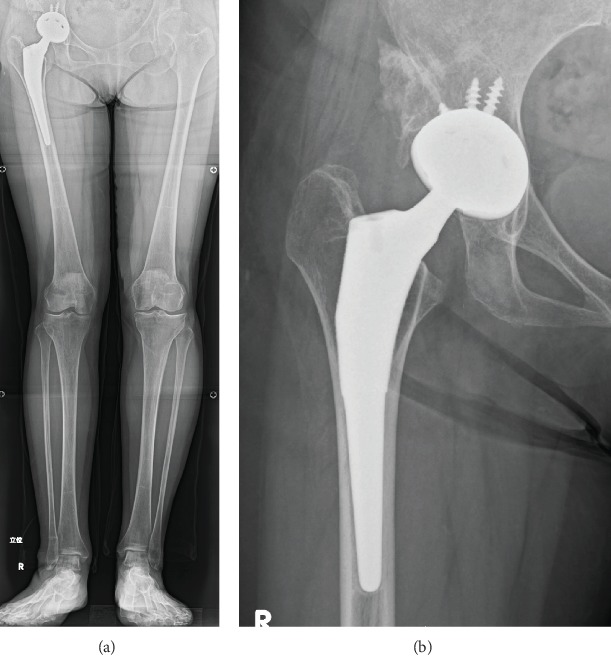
Anteroposterior radiograph of bilateral whole lower limbs in standing (a) and right hip joint (b) six months after surgery.
